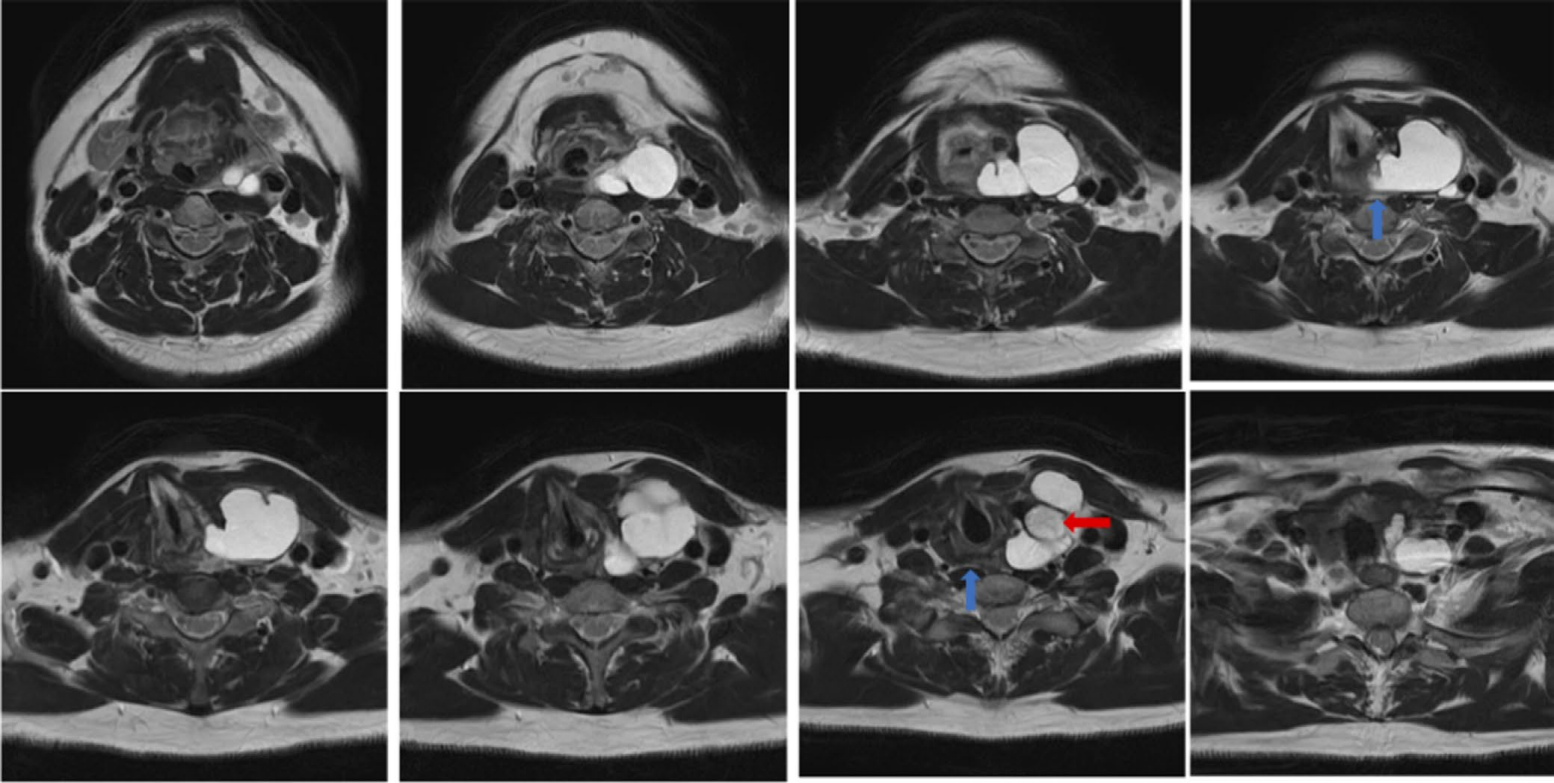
Axial MRI T1‐weighted images. The retropharyngeal space (RPS) was visualized as a linear layer of fat. The blue and red arrows indicate the RPS and primary tumor, respectively.
